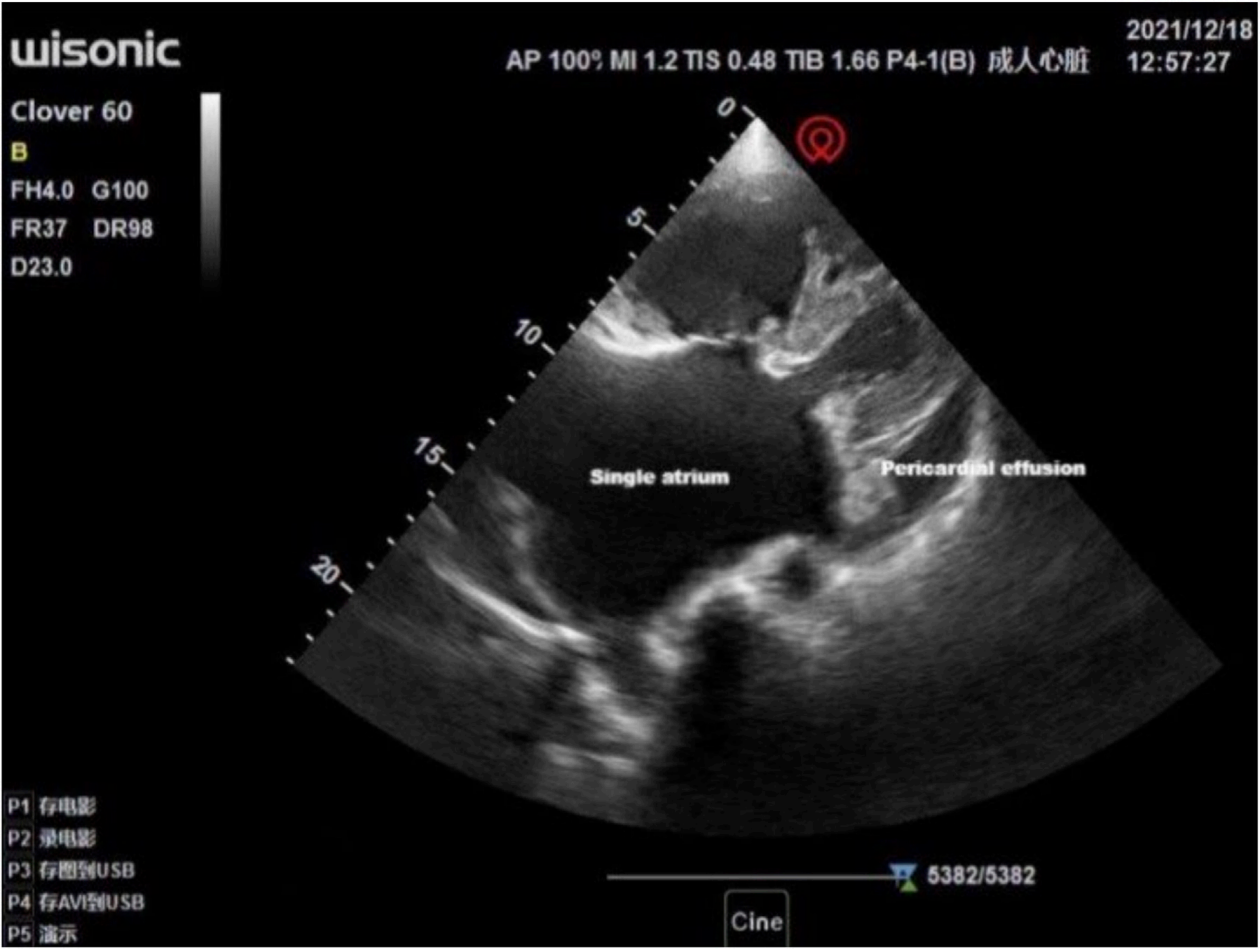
Visualization of the heart by standard transthoracic echocardiography was optimal from the apical views allowing to display a single atrium and moderate amount of pericardial effusion.
